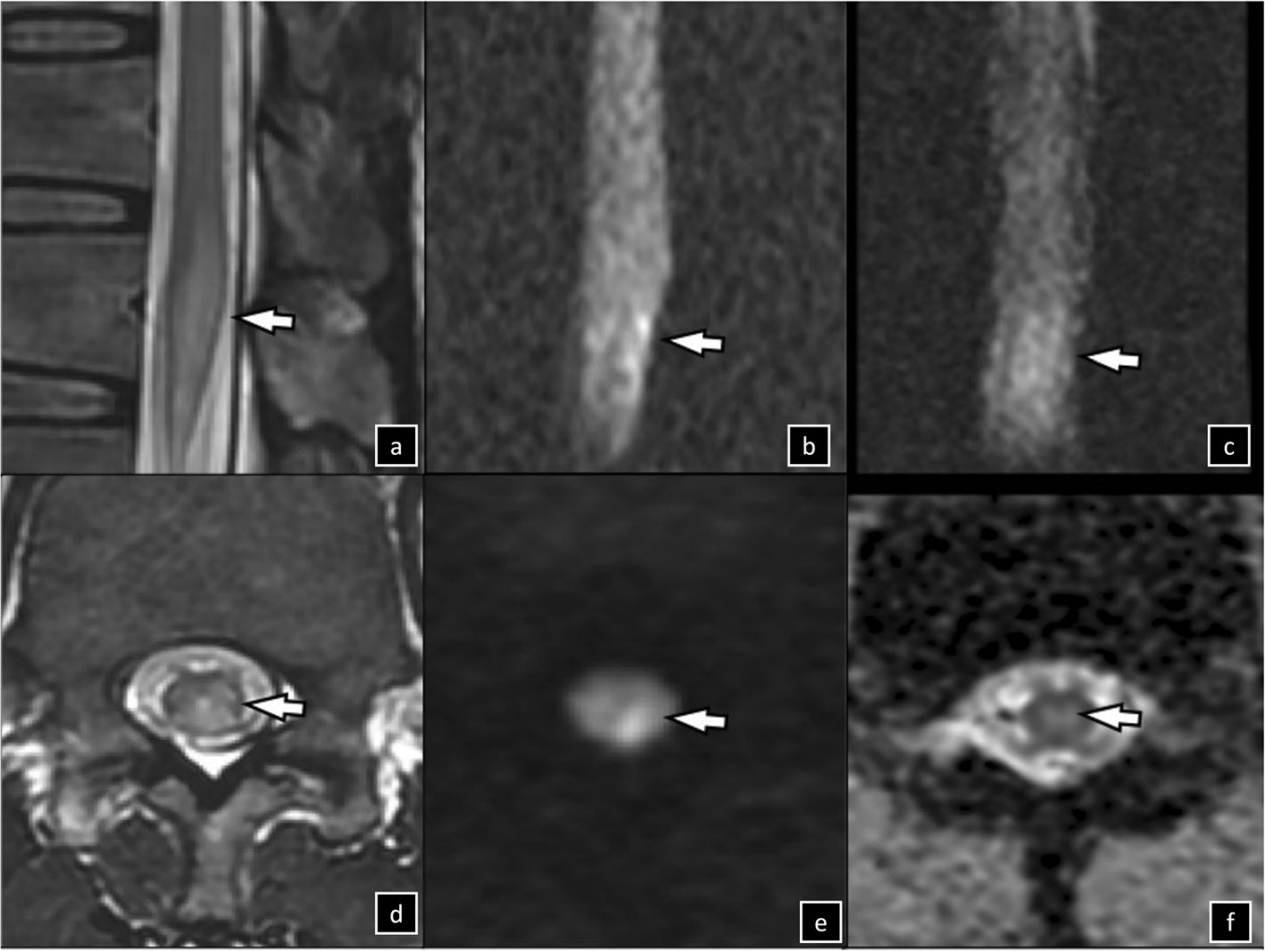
Spine MRI of patient 1, 9 days after symptoms onset. High signal intensity of sagittal (a, b, c), axial (d, e, f), T2-weighted (arrows on a, d), diffusion-weighted (arrows on b, e) images; subtle hyperintensity of sagittal (arrow on c) and axial apparent diffusion coefficients (arrow in f) were noted at levels T11–12. MRI, magnetic resonance imaging
